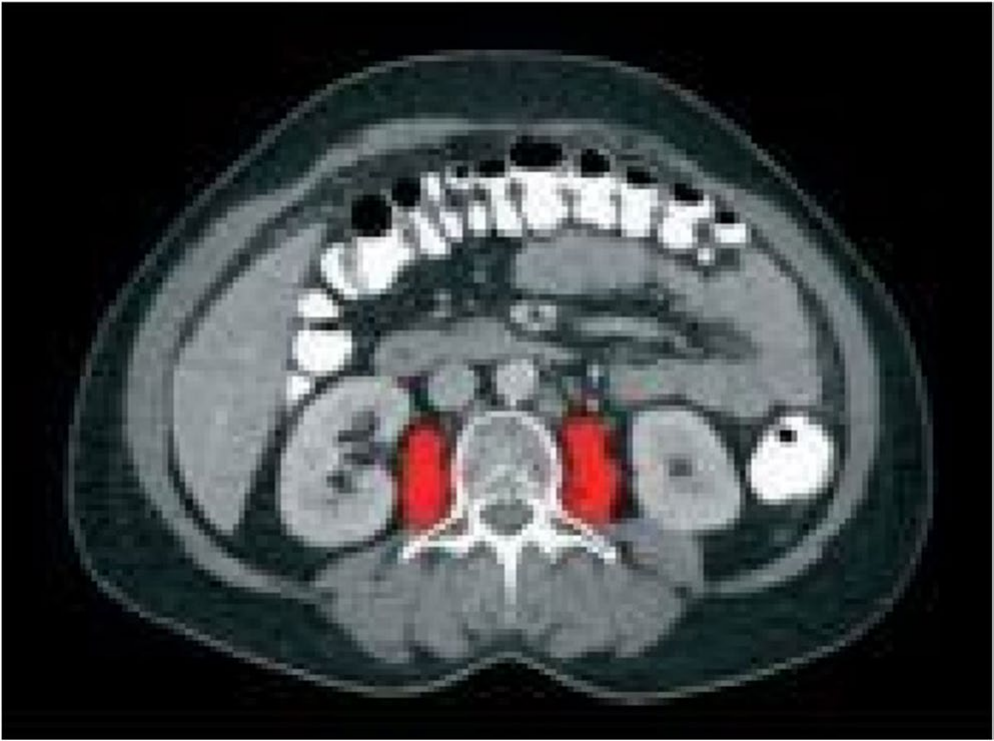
Psoas muscle area, Red painted (Abdominal CT, Axial Section, L3 level)
